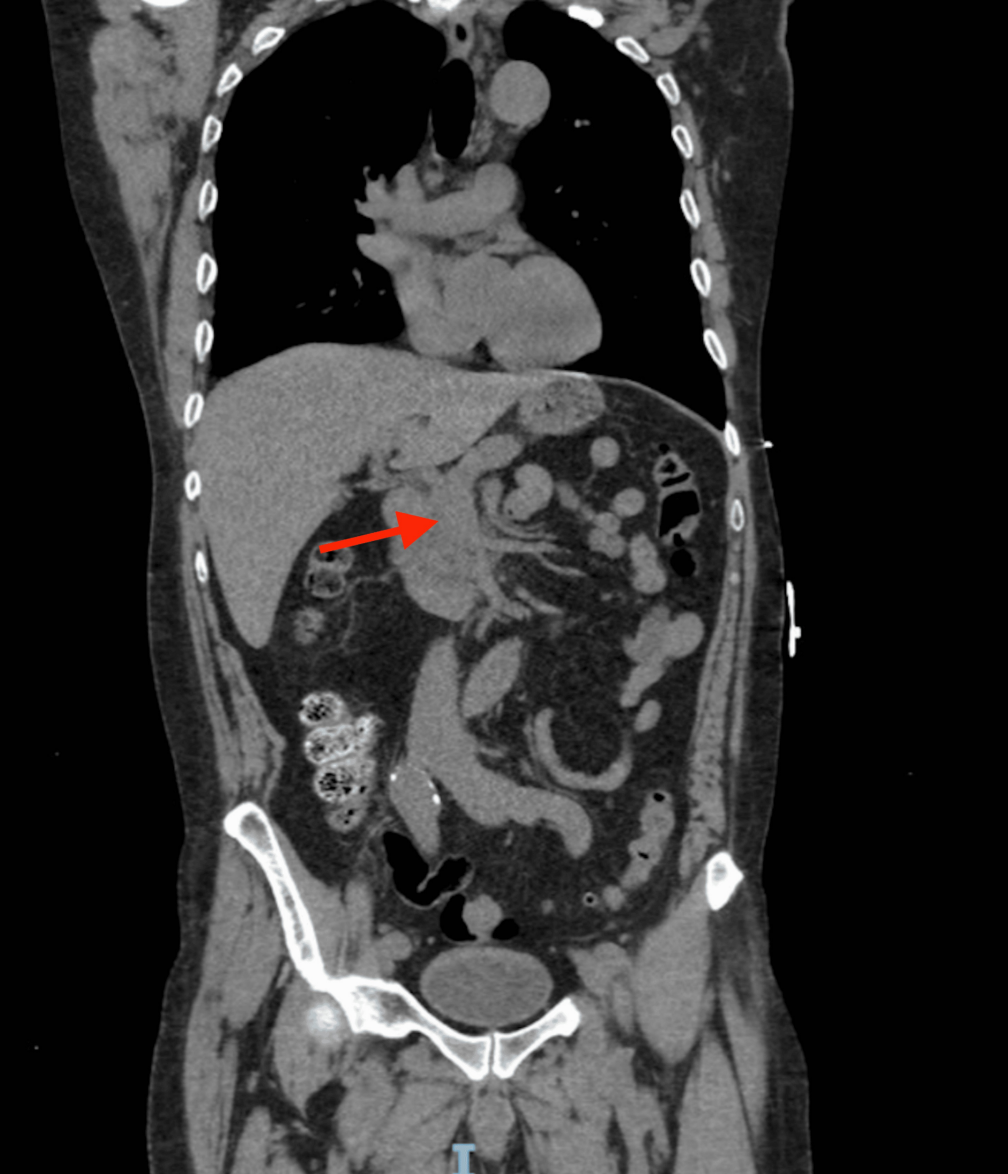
Coronal reformatted CT angiogram showing dissection of the proximal celiac artery This coronal reformatted CT angiogram shows dissection of the proximal celiac artery (arrow). The dissection flap is seen extending from the celiac origin without evidence of extension into the aorta or superior mesenteric artery. Surrounding organs are well perfused, with no signs of bowel ischemia or infarction.
